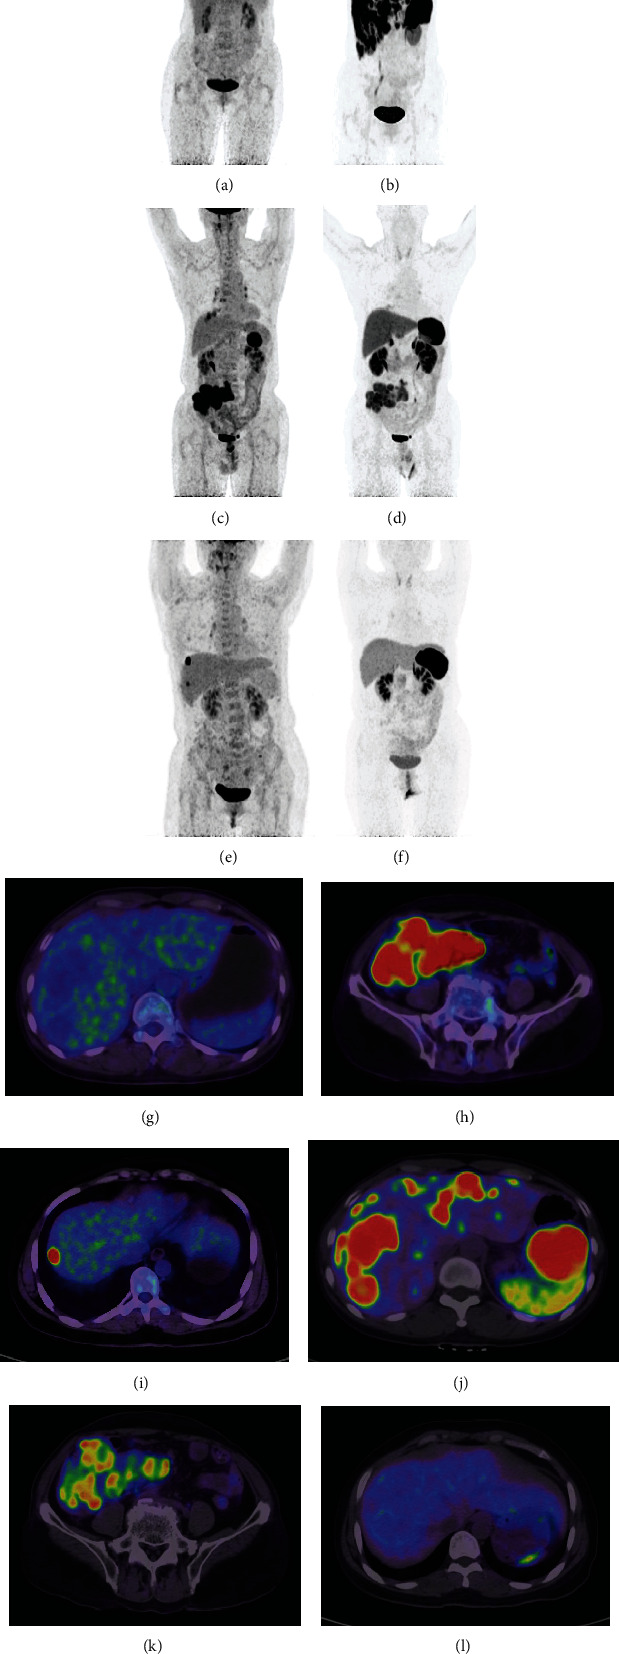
Shows the performance of ^18^F-FDG and ^18^F-OC in three patients. The maximum intensity projection (MIP, a–f) images from the respective PET datasets are shown. Images a, b, g, and j are from a patient with a G1 neuroendocrine tumor that had a NETPET score of P1, indicating that the scans are negative for ^18^F-FDG (a and g) and positive for ^18^F-OC (b and j) in the liver and abdominal cavity. Images c, d, h, and k are from a patient with a G3 neuroendocrine tumor of the ileocecum and lymph nodes and show that the lesions have greater avidity for ^18^F-FDG (c and h) than ^18^F-OC (d and k), with a NETPET score of P4b. Images e, f, i, and l are from a patient after rectal G3 neuroendocrine tumor surgery that had a NETPET score of P5, indicating that the scans are negative for ^18^F-OC (f and l) and positive for ^18^F-FDG (e and i) in the liver.
